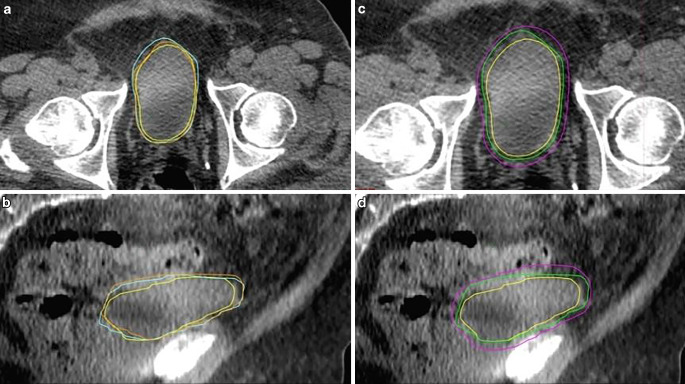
Creating a patient-specific margin. a,b Bladder contours: *yellow* CBCT 1, *orange* CBCT 2, *blue* CBCT 3. c,d
